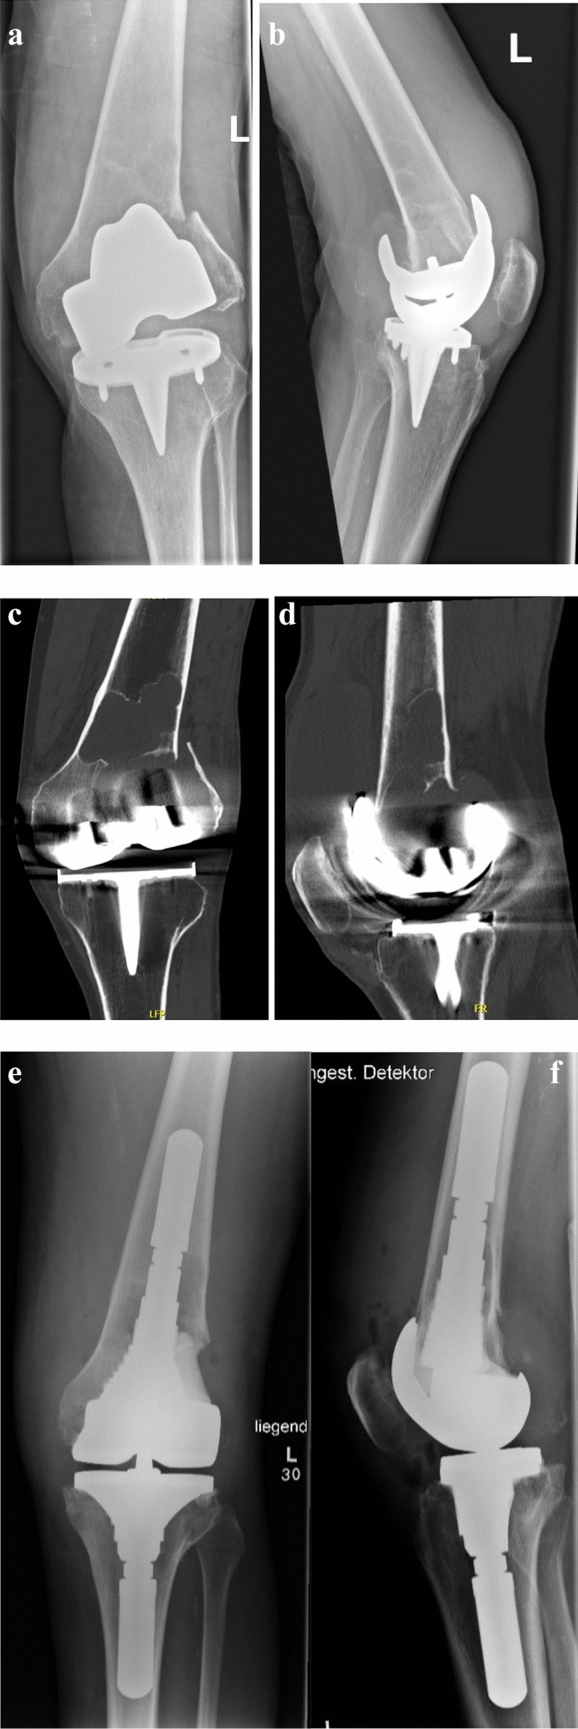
a Sixty-nine-year-old male patient with a dislocated periprosthetic distal femur fracture type Lewis–Rorabeck III. Technique: AP X-ray of the left knee joint. The femoral component seems loose and is dislocated. b Lateral X-ray of the knee joint. The distal femur shows multiple cystic lesions. c, d Digitally reconstructed computed tomography scan of the left knee joint in anterior and lateral views. The CT scan confirmed the loss of the femoral component and massive cystic formations in the proximal femur. Postoperative AP X-ray (e) and lateral view (f). The loosened knee replacement was removed and replaced with a cemented revision prosthesis (MUTARS^®^ GenuX^®^, Implantcast GmbH, Buxtehude, LS, Germany). The lateral fragment could not be refixed and was removed
